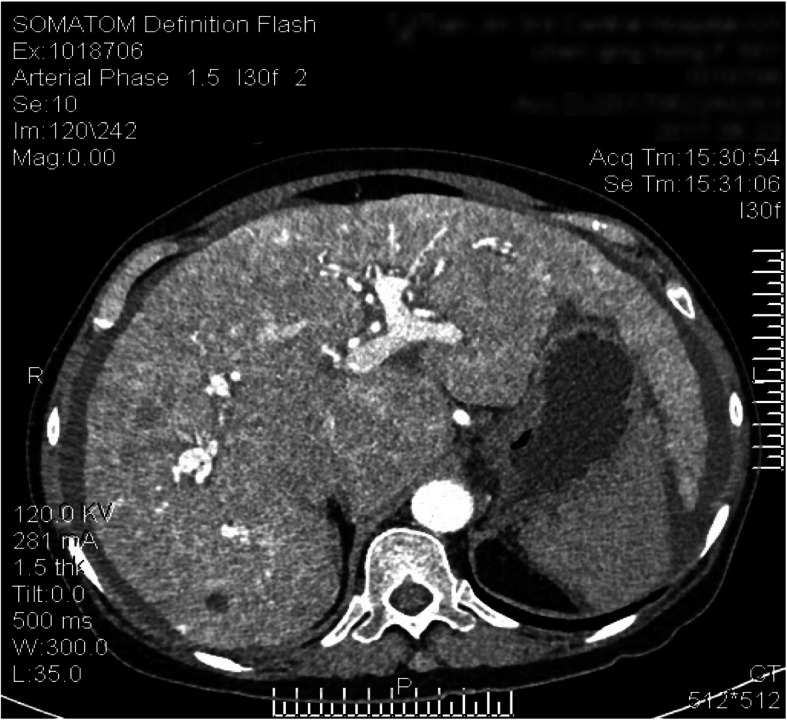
Contrast-enhanced spiral CT of the upper abdomen: This contrast-enhanced CT image shows the portal vein, enhanced liver parenchyma in the arterial phase, and multiple arteriovenous fistulae
